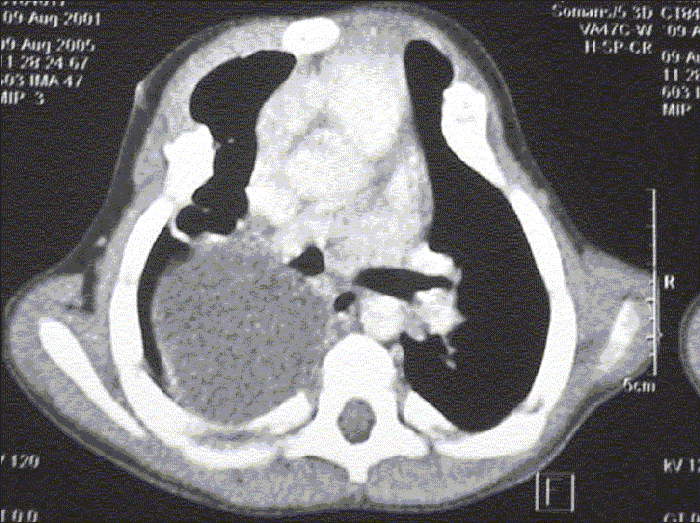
CT scan chest showing well defined posterior mediastinal cyst
